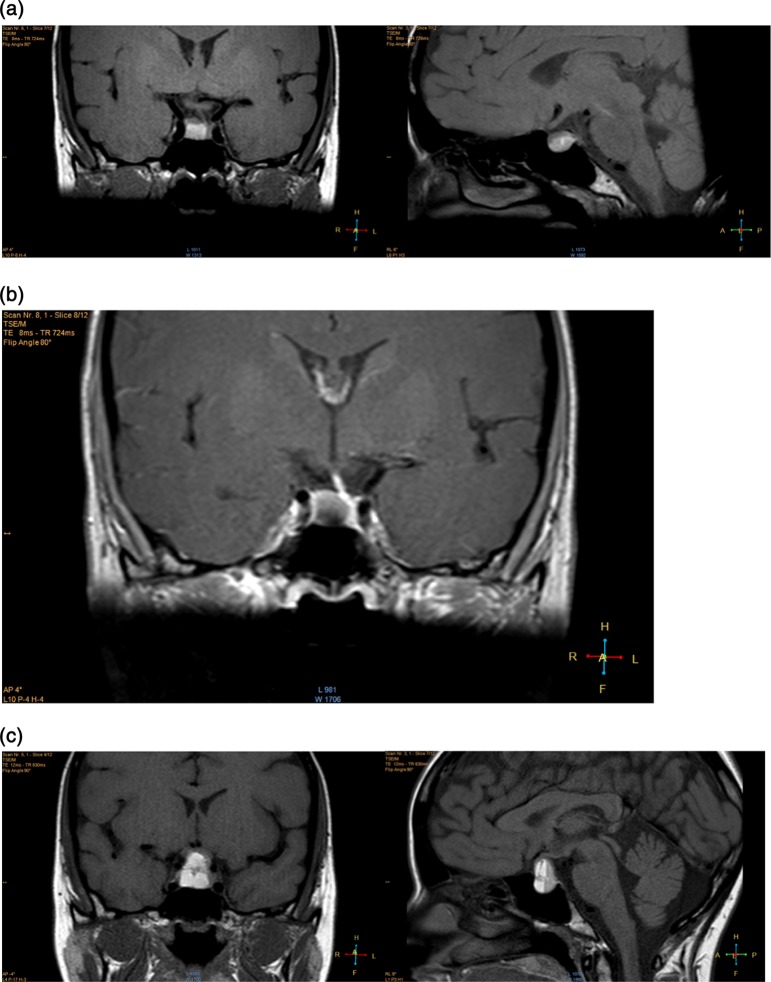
(a) Sagittal and coronal T1-weighted MRI scans showing cystic lesion in the central and right side of pituitary, consistent with microadenoma (6 mm in height and 9–10 mm transversely). The infundibulum is deviated to the left. (b) Gadolinium-enhanced T1-weighted coronal pituitary MRI; (c) sagittal and coronal T1-weighted MRI showing pituitary haemorrhage with a significant interval increase in the size of the adenoma (16–17 mm in height) and optic chiasmal compression.
